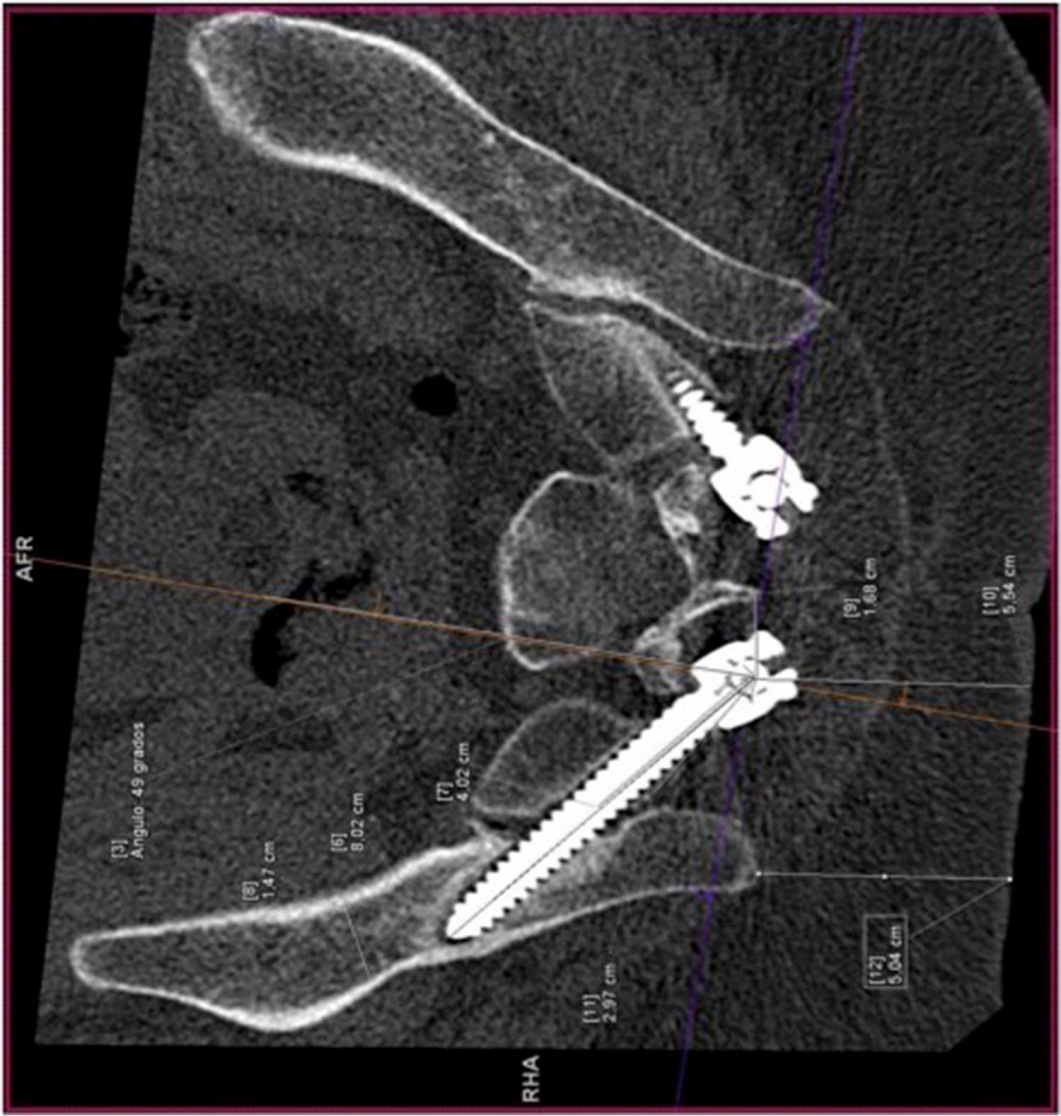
Ideal trajectory. CT imaging plane (projection of the line between PSIS and the anterior inferior iliac spine (AIIS)). The ideal trajectory is the line drawn perpendicular to the narrowest region
